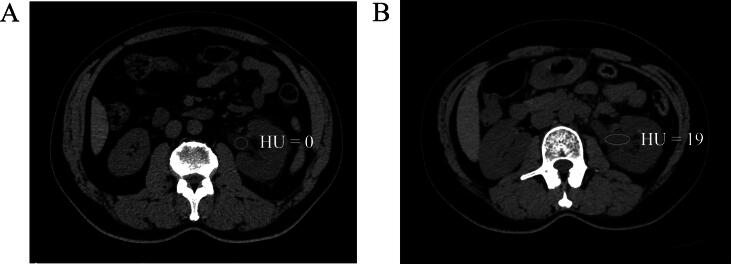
Examples for the measurement of renal pelvis urine by NCCT: (A) an example of the lower value of HU in renal pelvis urine in the patient who was free of severe infectious complications after ureteroscopy and (B) an example of the higher value of HU in renal pelvis urine in the patient who experienced severe infectious complications after ureteroscopy. HU = Hounsfield unit; NCCT = non–contrast-enhanced computed tomography.
